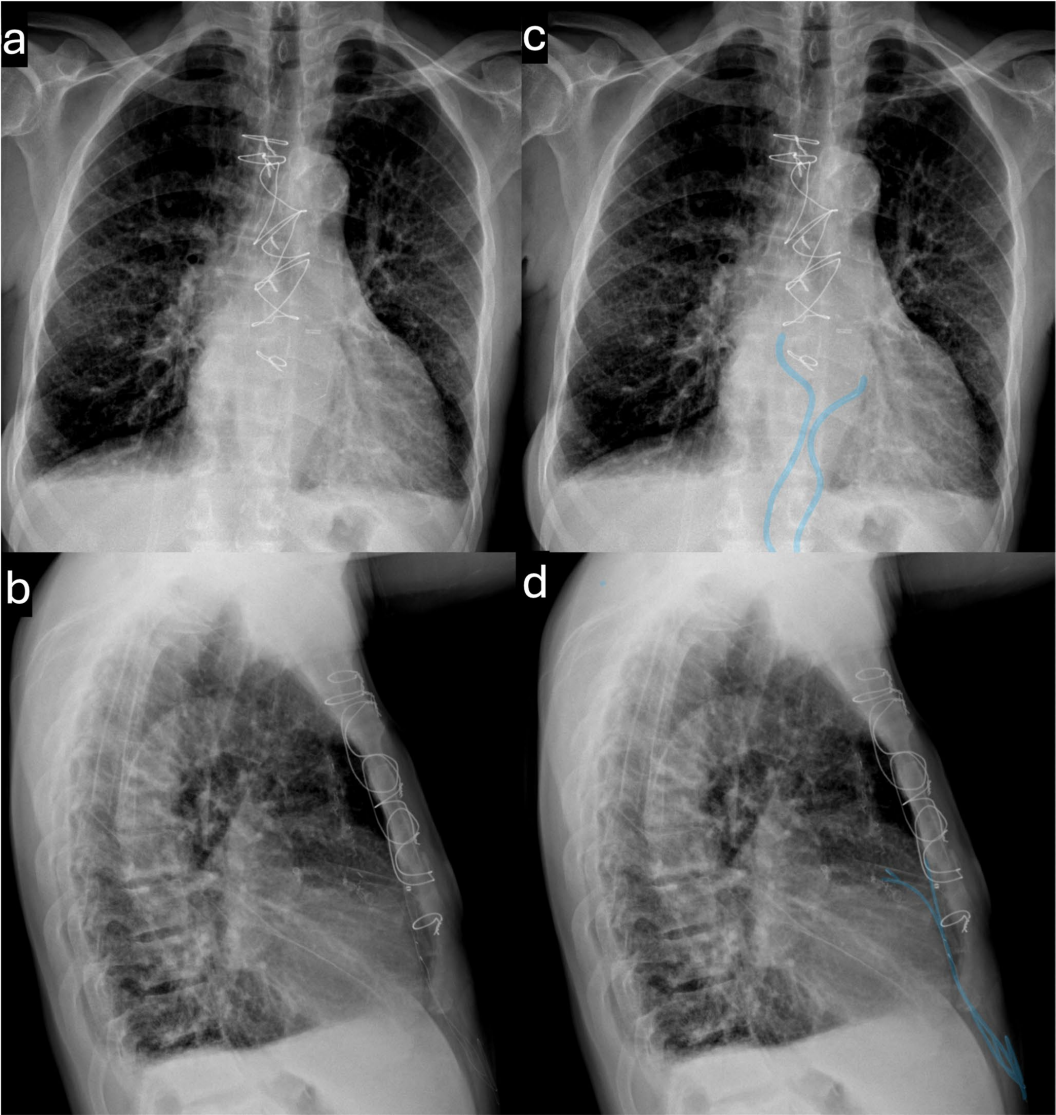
Chest X-rays displaying abandoned epicardial pacing wires. Panels a and b show the frontal and lateral views, respectively, without annotations. Panels c and d highlight the epicardial pacing wires, marked with blue lines, on the corresponding frontal (c) and lateral (d) chest X-rays
